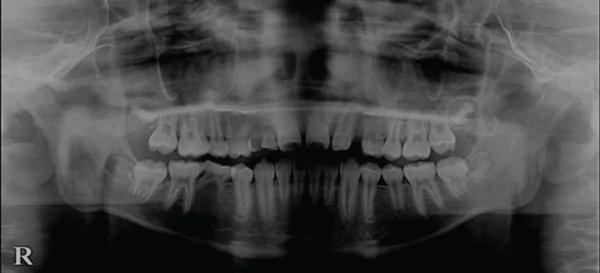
Panoramic radiograph showing absence of the permanent tooth germs at FDI 12, 13, 23, and 45.
